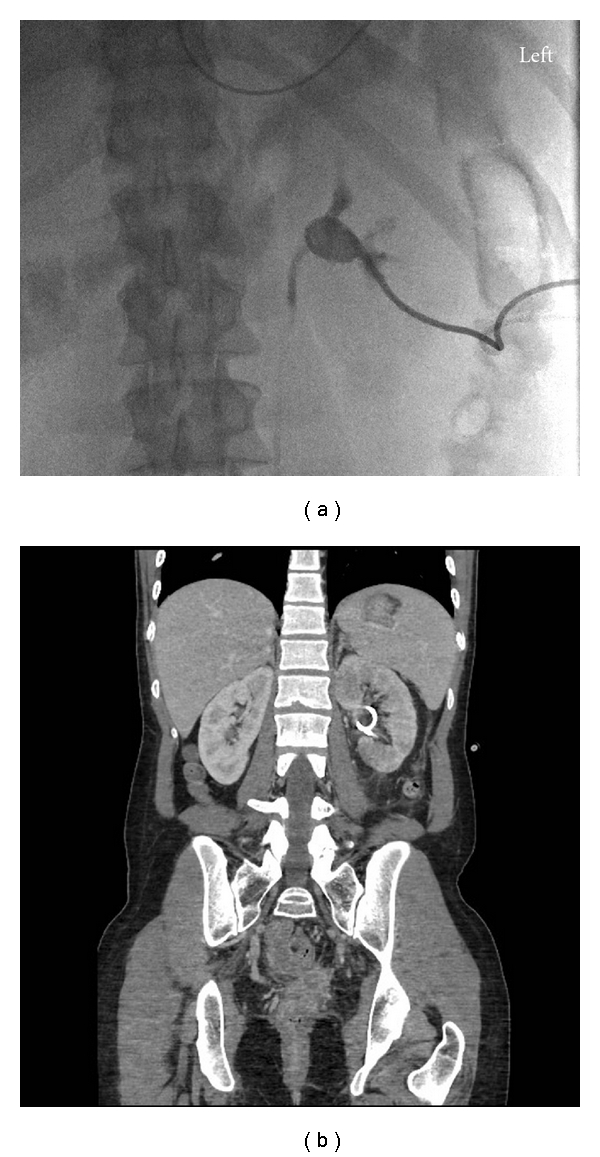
21-year-old woman with cervical cancer develops ureteric obstruction due to local disease invasion. (a) Contrast injection immediately following percutaneous nephrostomy confirms 8 french catheter in good position within renal collecting system. (b) Contrast-enhanced CT scan confirms percutaneous nephrostomy catheter in positioned within left renal collecting system. Patient is post total abdominal hysterectomy and bilateral salpingo-oophorectomy.
